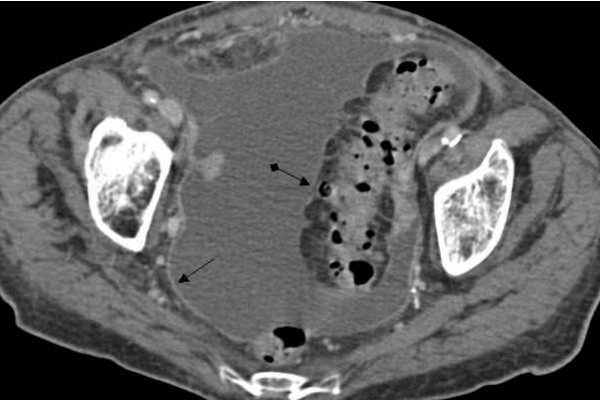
CT pelvis pronounced contrast enhancement of the peritoneum (); thickened wall of the sigmoid colon ().
